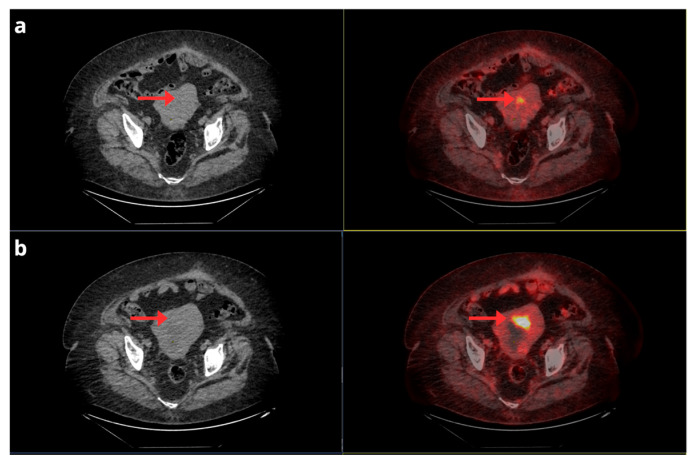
A 65-year-old female with a history of stage IB FIGO serous EC, treated with radiotherapy and brachytherapy. A comparison of two [^18^F]F-FDG PET/CT studies is presented. The axial fused CT and PET/CT slices in the top row correspond to the first post-treatment study, showing residual focal uptake in the anterior uterine wall (a). The axial fused CT and PET/CT slices in the bottom row, from the follow-up study 6 months later, demonstrate an increase in both the intensity and extent of this uptake suggestive of progression (b).
